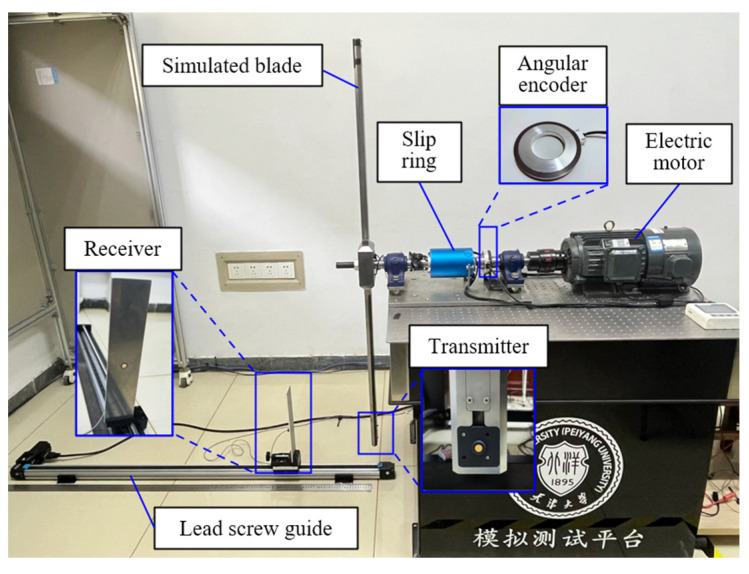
The experiment platform of ultrasonic dynamic measurement.
